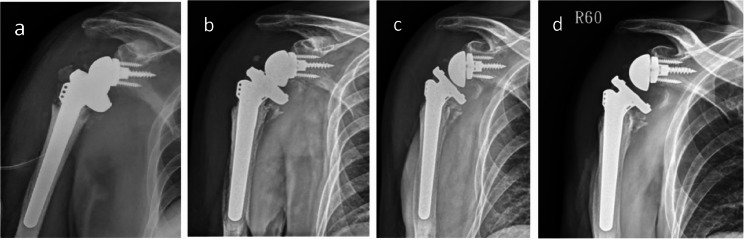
Case 1. a Third time open reduction. b, c, d X-ray after 6 months, 1 year, 1.5 years respectively
